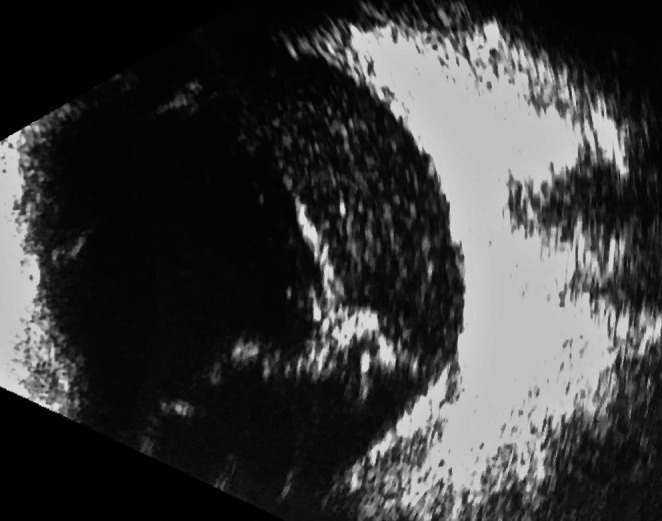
B‐scan ultrasonography of the left eye. B‐scan ultrasonography revealed mobile hyperechoic vitreous opacities and membrane formation.
